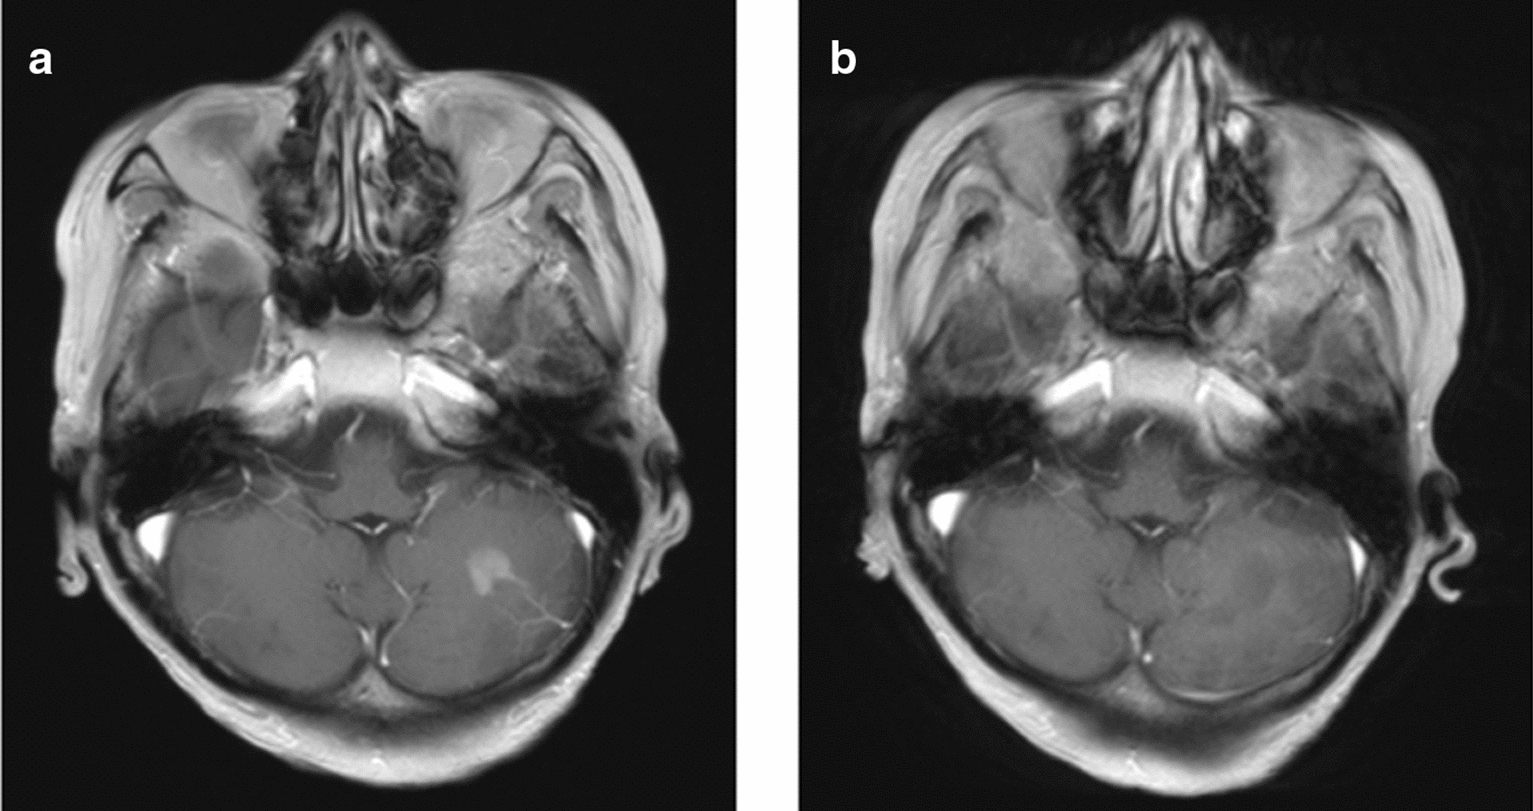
Changes of radiological appearance of the lesion on magnetic resonance imaging before and after tirabrutinib treatment are shown. The lesion was initially enhanced by a contrast agent in the left cerebellum before tirabrutinib treatment (a), but the enhanced lesion disappeared one month after tirabrutinib treatment (b)
